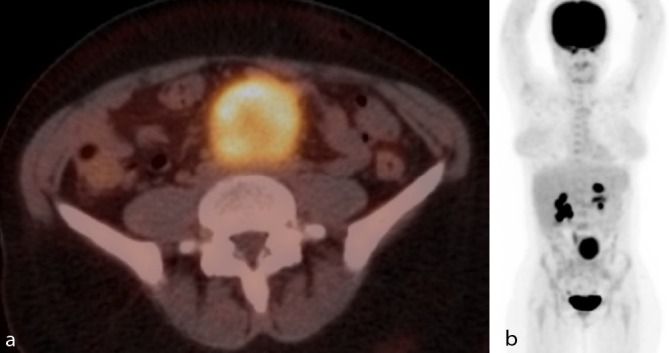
Axial ^18^F-FDG-PET CT image (a) and coronal maximal-intensity-projection ^18^F-FDG-PET image (b). There is a large, solitary and well-defined mass with marked avidity of ^18^F-fluorodeoxyglucose. There are no distant ^18^F-FDG-avid locoregional lymph nodes or masses.
